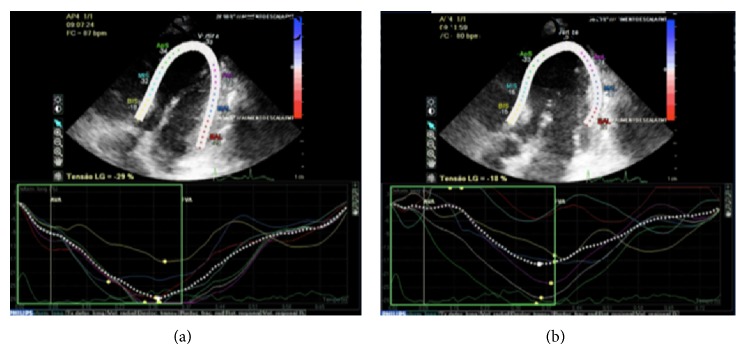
Speckle tracking echocardiogram with Doppler. Comparison between images during and after dobutamine infusion ((a) and (b)), showing differences in systolic global strain (29% and 18%, resp.).
